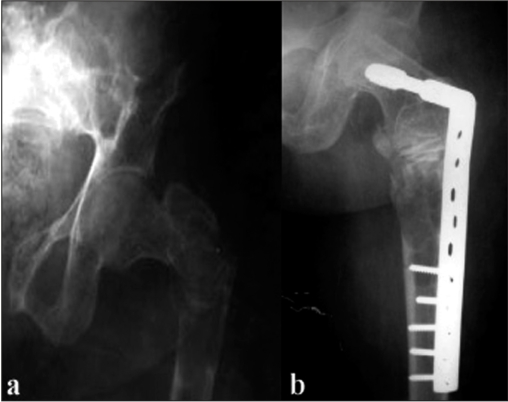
(a) Pre-operative anteroposterior radiograph showing pathological subtrochanteric fracture. (b) Postoperative follow-up anteroposterior radiograph showing DCS fixation with fibular and cortico cancellous grafts *in situ*
